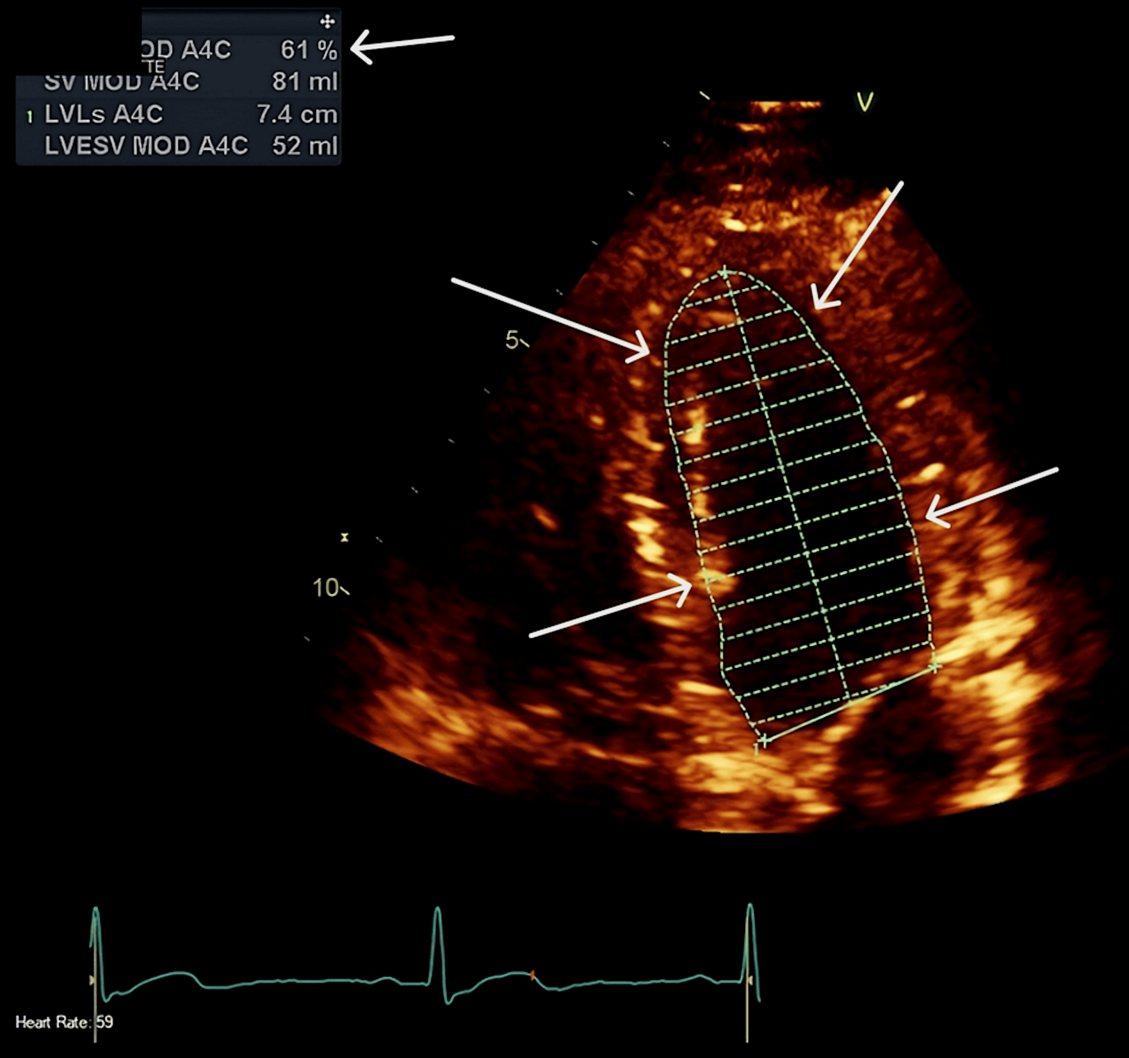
2D TTE Day 10 showing improvement of left ventricular ejection fraction to 61%. Arrows delineating the left ventricular internal borders used to calculate the ejection fraction of 61% by Simpson's biplane. TTE: transthoracic echo
